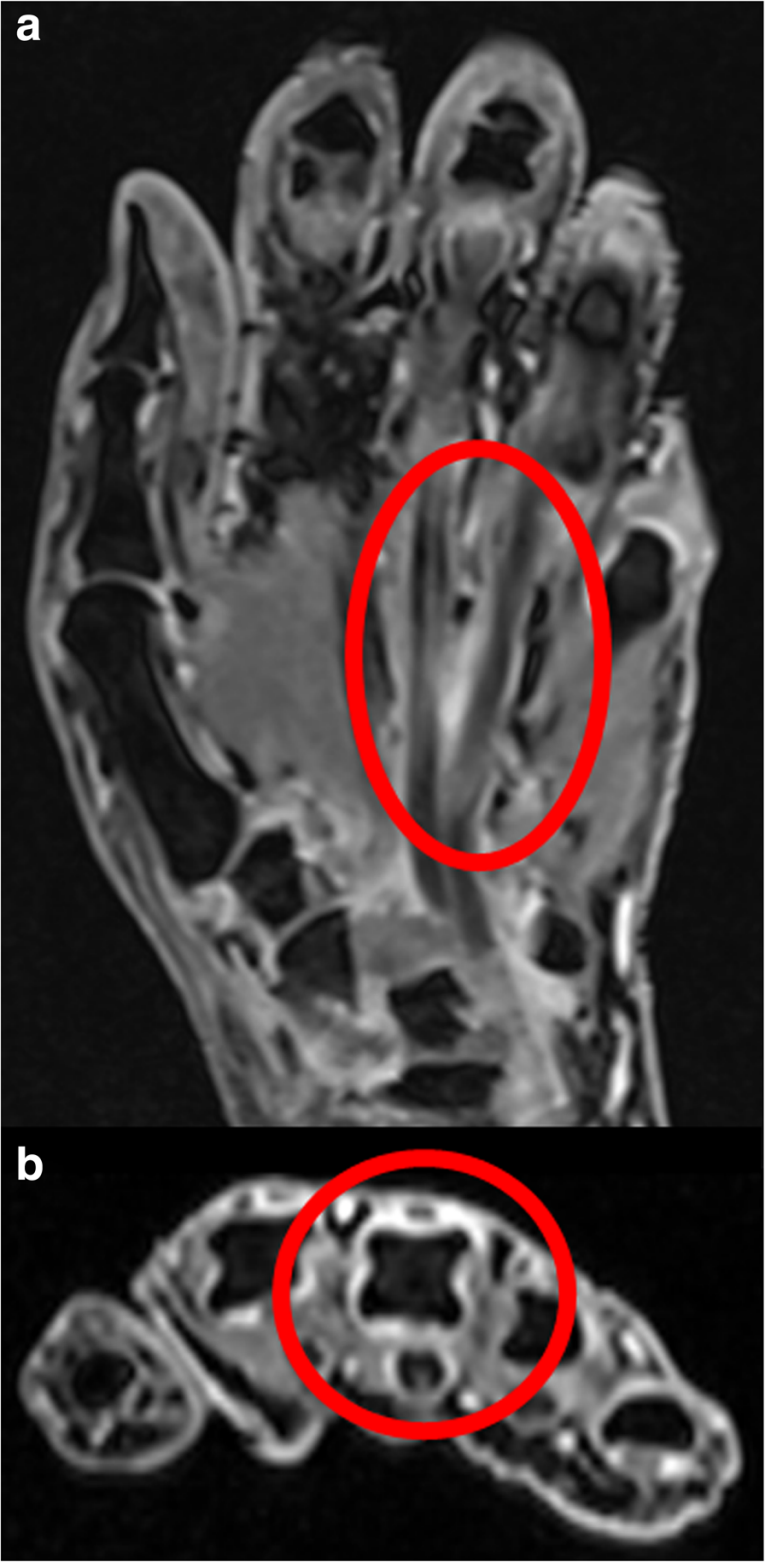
Contrast magnetic resonance imaging of the patient’s right hand. Synovial hyperplasia presented as a low intensity signal around the carpal bone (a) and fingers (b). A contrast enhancement effect was seen with gadolinium (red circles)
